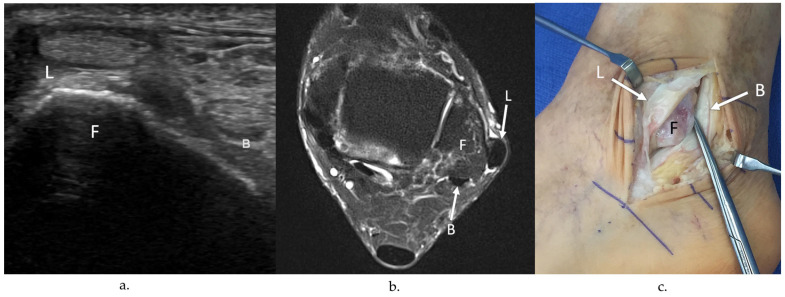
(a) Transverse (short axis) grayscale US image of the left ankle shows anterior dislocation of the peroneus longus tendon (L) from the lateral retromalleolar groove (F), secondary to a SPR tear. The peroneus brevis tendon (B) is normally located in the retromalleolar groove. (b) Axial T2-weighted MR image with fat saturation of the same ankle confirms anterior dislocation of the peroneus longus tendon (L) from the retromalleolar groove of the fibula (F) with normally located PB tendon (B). (c) Intraoperative image confirms anterior dislocation of the peroneus longus (L) tendon, secondary to a SPR tear. Note the normal retromalleolar location of the peroneus brevis tendon (B).
